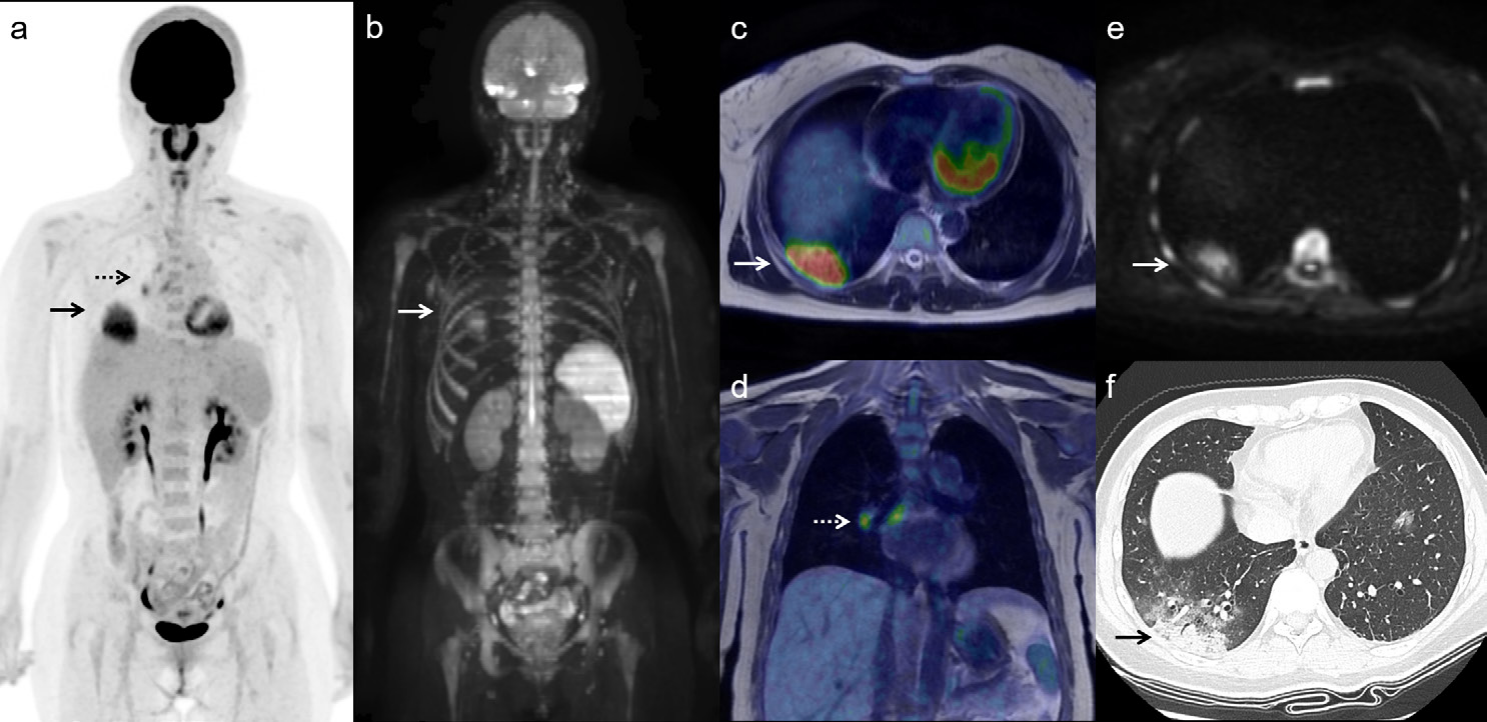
Maximal intensity projection images of FDG PET (a) and DWI
